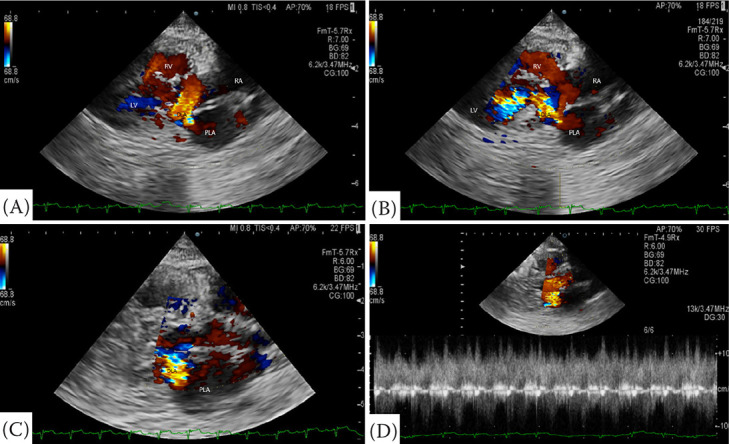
Colour-flow Doppler and continuous Doppler echocardiography images of the right parasternal 4-chamber view during systole and diastole (A) During systole, blood flow from the left atrium to the right atrium was confirmed through a defect in the atrial septum. (B) During diastole, blood flow from the left atrium to the left ventricle was observed. (C) Turbulent continuous blood flow was revealed in the distal left atrium chamber regardless of systole or diastole. (D) Turbulent continuous blood flow velocity was measured at 1.3 m/s with a pulsed-wave Doppler
